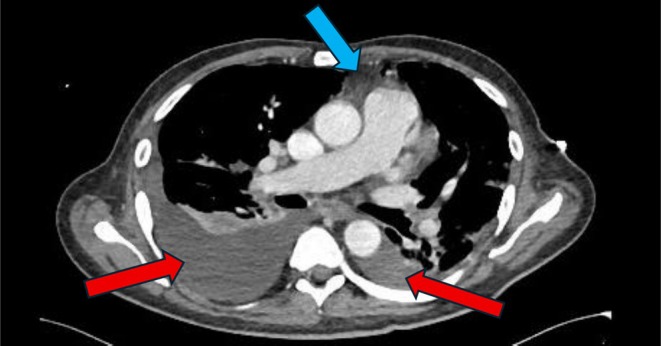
Thorax CT anterior mediastinal mass as described above, possibly representing thymus gland tumor (blue arrow), bilateral pleural effusion with associated lung collapse/consolidation more on the right side (red arrows).
